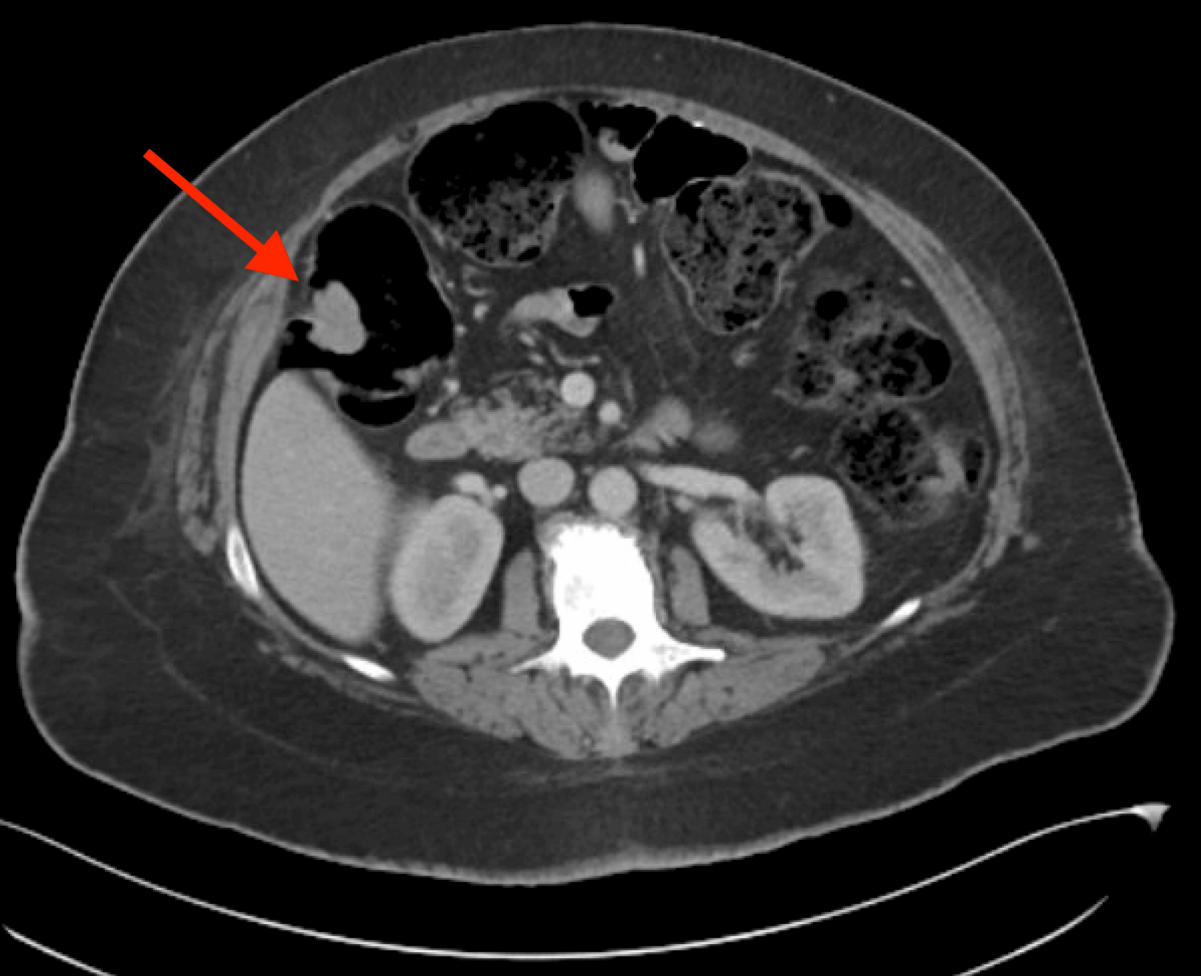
The CT scan of the abdomen and pelvis revealed a lobulated polypoid lesion in the hepatic flexure, measuring 2.9 x 1.7 x 2.8 cm (red arrow).
